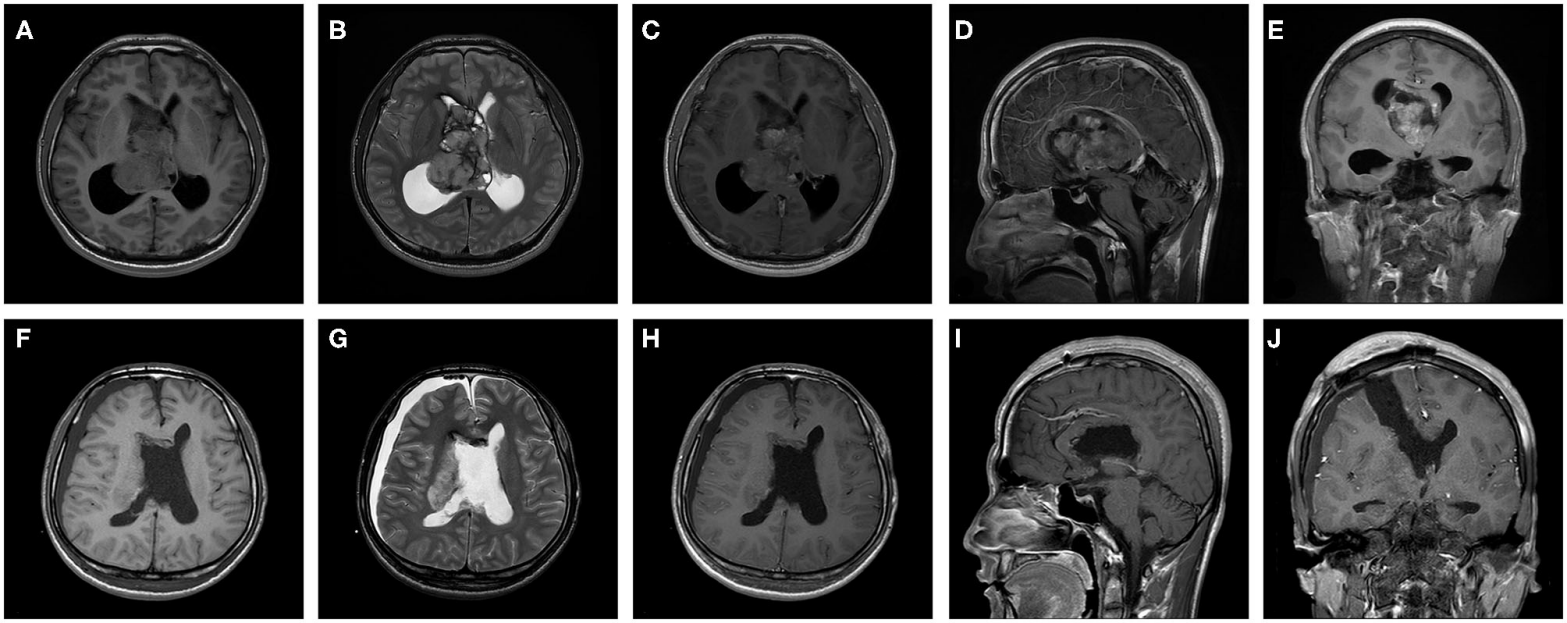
A 27-year-old male patient with central neurocytoma in treatment with surgery. Preoperative (A) T1 weighted axial sequence, (B) T2-weighted axial sequence, (C) T1- enhanced axial sequence, (D) T1-enhanced sagittal sequence, and (E) T1-enhanced coronal sequence MRI scans showing a 64.7 × 44.8 × 49.4 mm-sized mass in bilateral lateral ventricles and third ventricle and marked ventricular dilatation. Postoperative (F) T1 weighted axial sequence, (G) T2-weighted axial sequence, (H) T1-enhanced axial sequence, (I) T1-enhanced sagittal sequence and (J) T1-enhanced coronal sequence MRI scans indicating that CN was completely excised and ventricles return to normal slightly.
